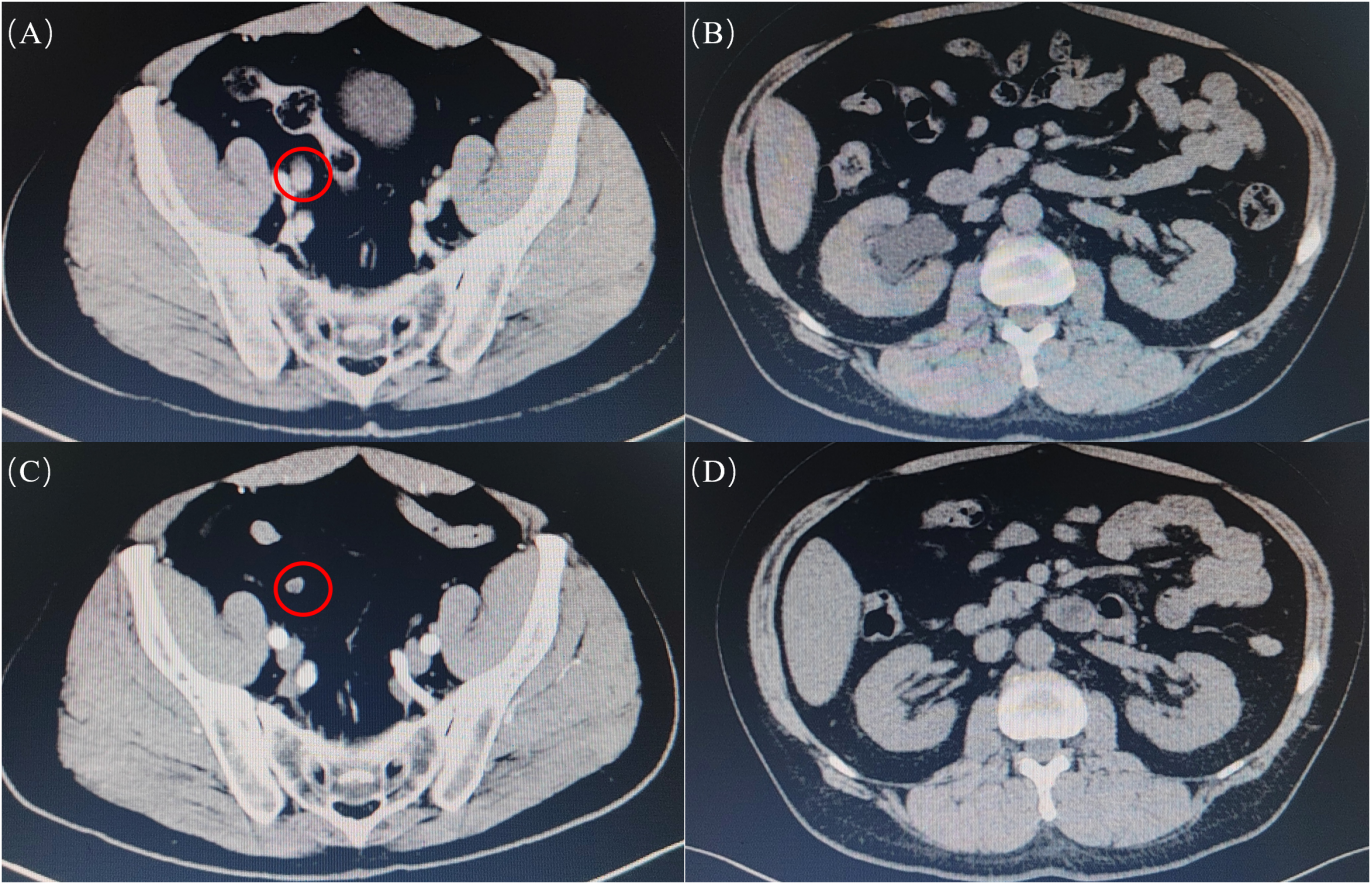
Preoperative and postoperative CT images of SU patients were received. (A) Preoperative ureteral tumor site of the patient; (B) Preoperative hydronephrosis; (C) No recurrence was found in the operation area of the same patient 19 months after operation; (D) No hydronephrosis was found in the same patient 19 months after operation.
